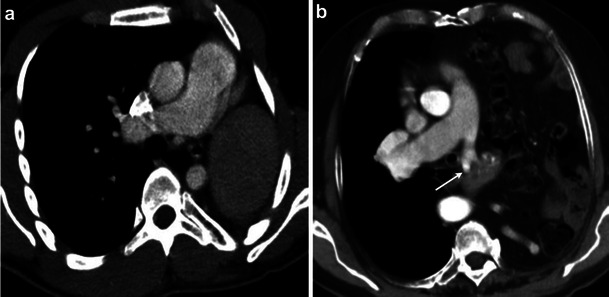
Contrast-enhanced CT image (a) shows complete agenesis of left lung and left pulmonary artery. The left hemithorax is smaller with mediastinal shift toward the left and the elevation of the left hemidiaphragm. The abdominal contents are seen in the left hemithorax. Contrast-enhanced axial CT image (b) demonstrates partial agenesis of the left pulmonary artery (*arrow*) with hypoplasia of left lung. There is no mediastinal shift, but the abdominal organs extend into the thorax
